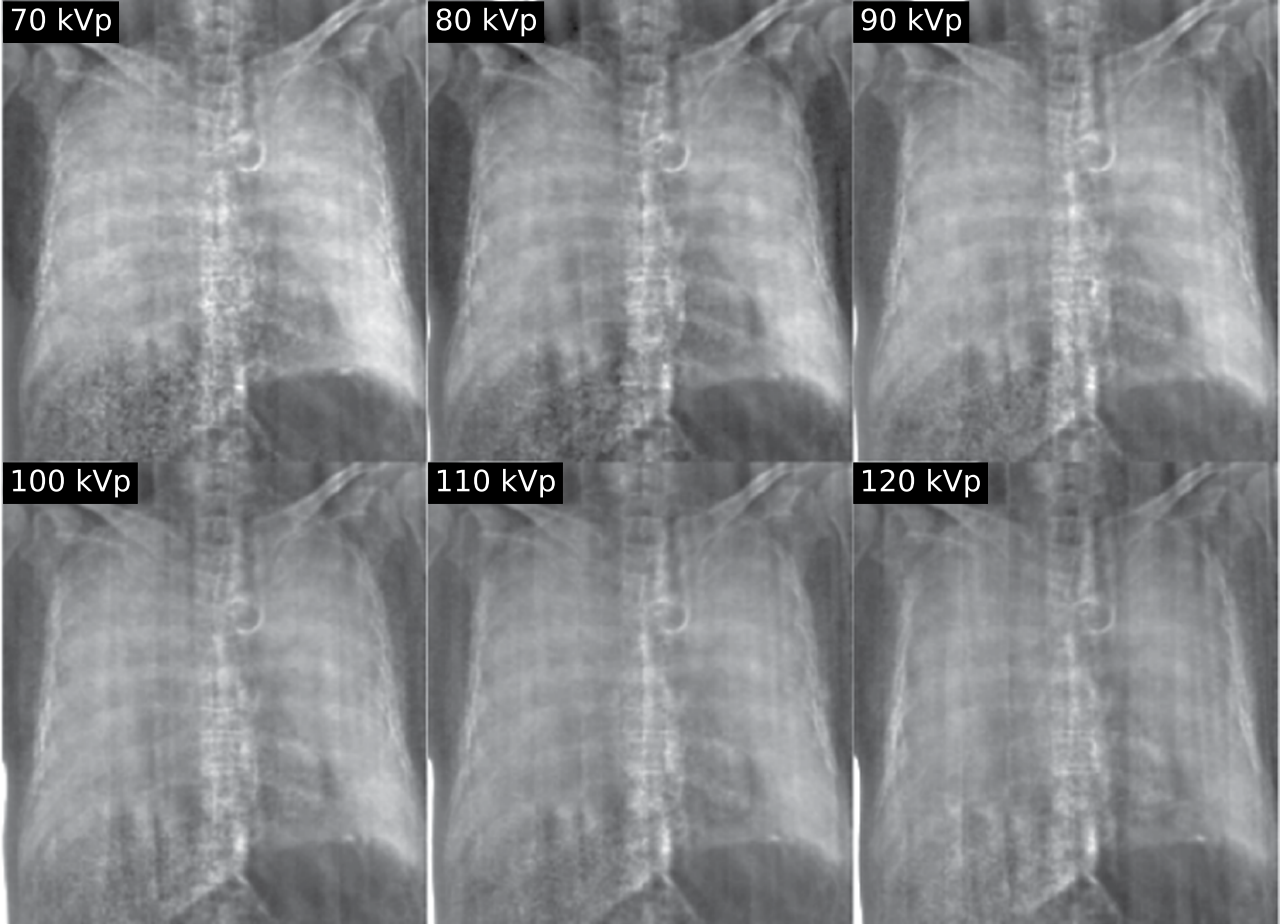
X-ray dark-field radiographs of a human thorax at the examined tube voltages. All images presented here and in Fig. 1a are shown with the same windowing and the same low-pass filter as in Fig. 1a was applied. The dark-field signal declines significantly toward higher tube voltages. Also, the image quality decreases with higher tube voltages due an increase of imaging artifacts.
